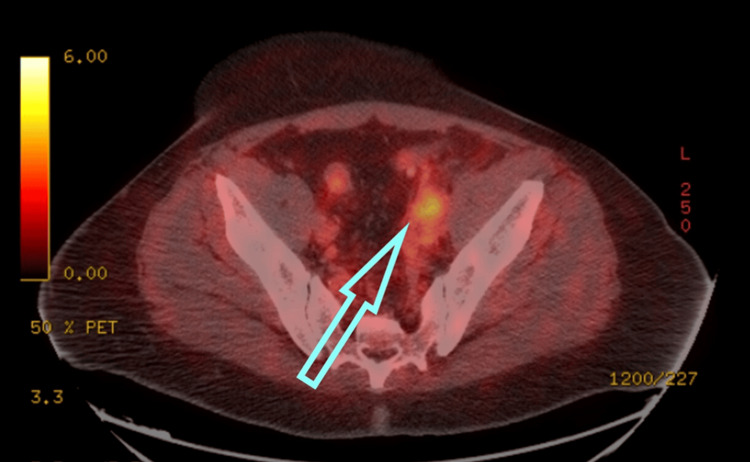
PSMA scan after the completion of the chemotherapy showing a decrease in the size of the psoas muscle mass, with residual metabolic activity (blue arrow), representing a positive response to the therapy. PSMA: prostate-specific membrane antigen
